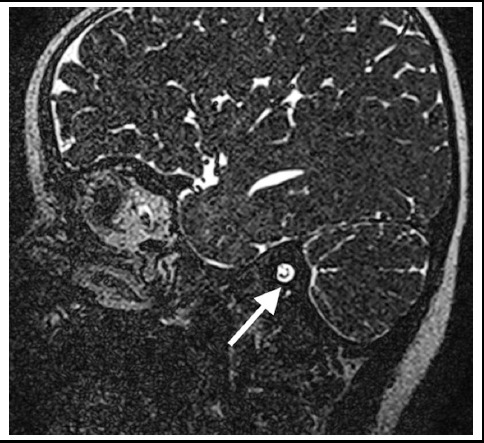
Thin-slice coronal computed tomographic image of a sample case with inner ear malformation obtained before cochlear implantation surgery demonstrating cochlear nerve in the internal acoustic channel included in the 4 nerve combination.
